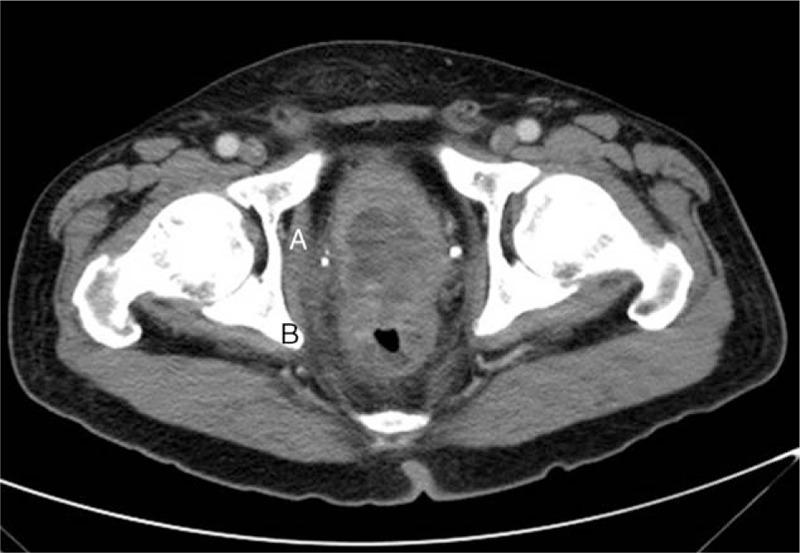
Computed tomography (CT) showed (A) a heterogeneous density of the prostate with central low attenuation, and (B) thickening of the rectum wall.
